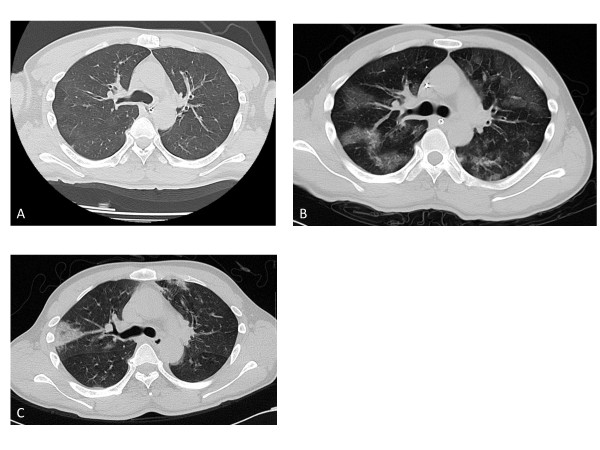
A typical case of SII chest CT. The BWT was 4.1 mm on admission (A), 4.4 mm after 24 hours (B), and 2.8 mm after 7 days (C). He had associated pneumonia and remained in the ICU for 14 days. SII, smoke-inhalation injury; CT, computed tomography; BWT, bronchial-wall thickness; ICU, intensive care unit.
